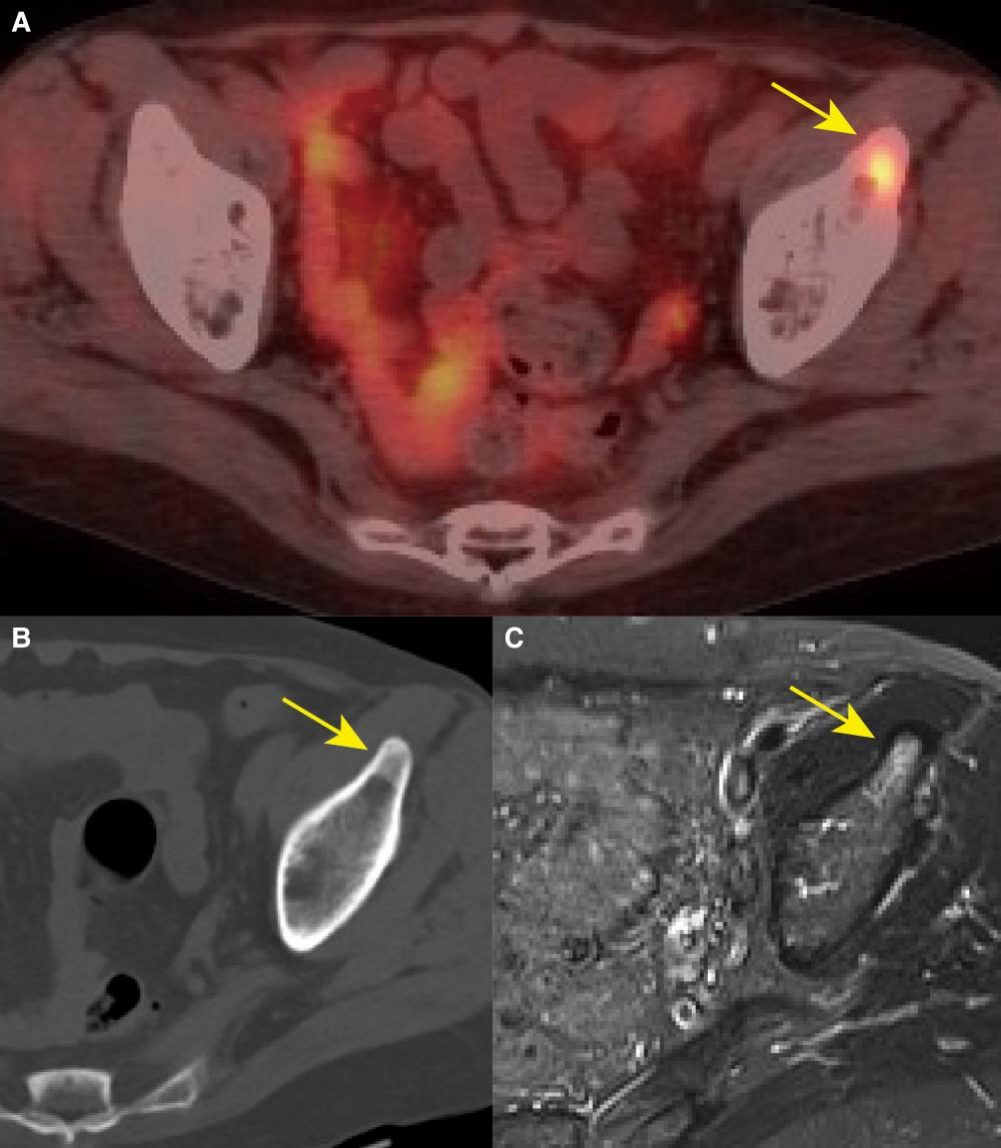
^68^Ga-DOTATOC PET/CT and pelvic CT and MR imaging. A, ^68^Ga-DOTATOC PET/CT: focal uptake in the left anterior inferior iliac spine (yellow arrow) clearly demonstrating the tumor location. B, CT imaging: A high-density area in the left anterior inferior iliac spine (yellow arrow). C, MR imaging: A high signal intensity of the left anterior inferior iliac spine on short tau inversion recovery image (yellow arrow).
